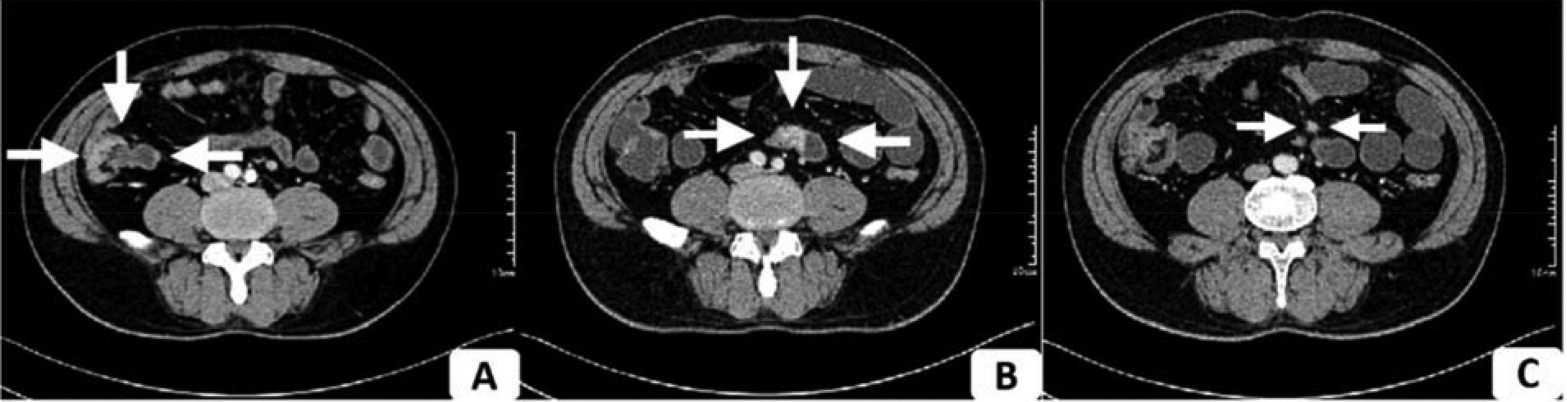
Abdominal thin-section contrast-enhanced CT. (A) Ileocecal intestine thickening (arrow). (B) Jejuno-ileum wall thickening and heterogeneous enhancement, with a 2.1 cm heterogeneously enhanced mass (arrow) obstructing the lumen. (C) Surrounding enlarged lymph node (arrow) and mesenteric kinks.
